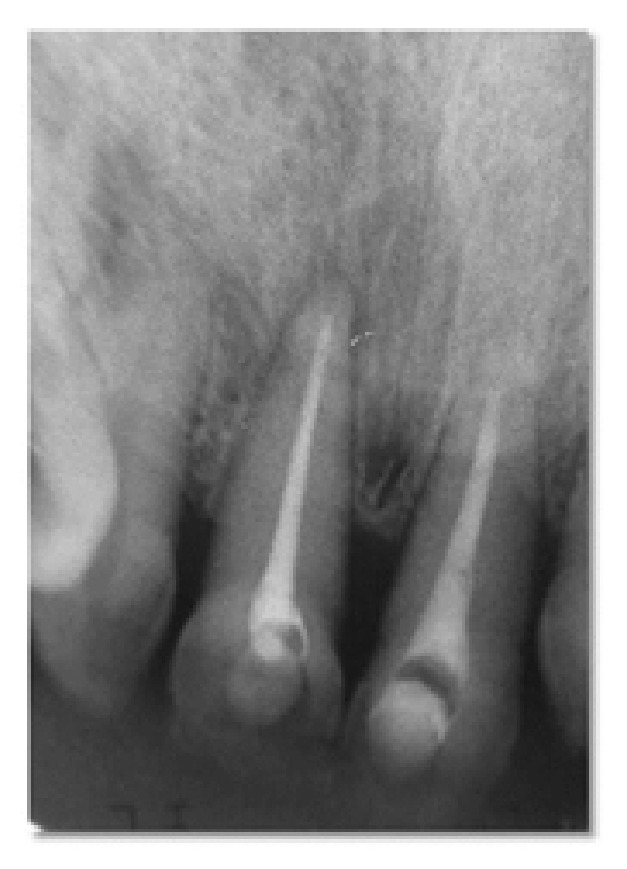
The radiographic view of the patient after root canal treatment.
